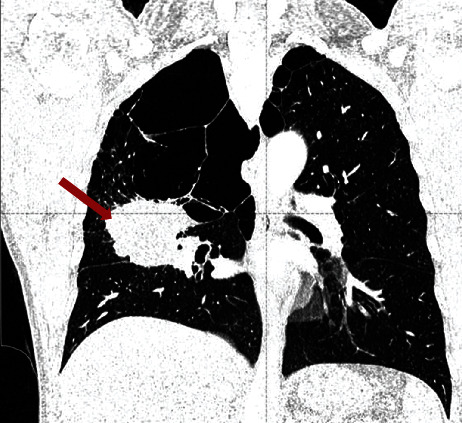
CT scan image of the right lung tumor (red arrow) extending to the three lobes with interbronchial lymph nodes.
